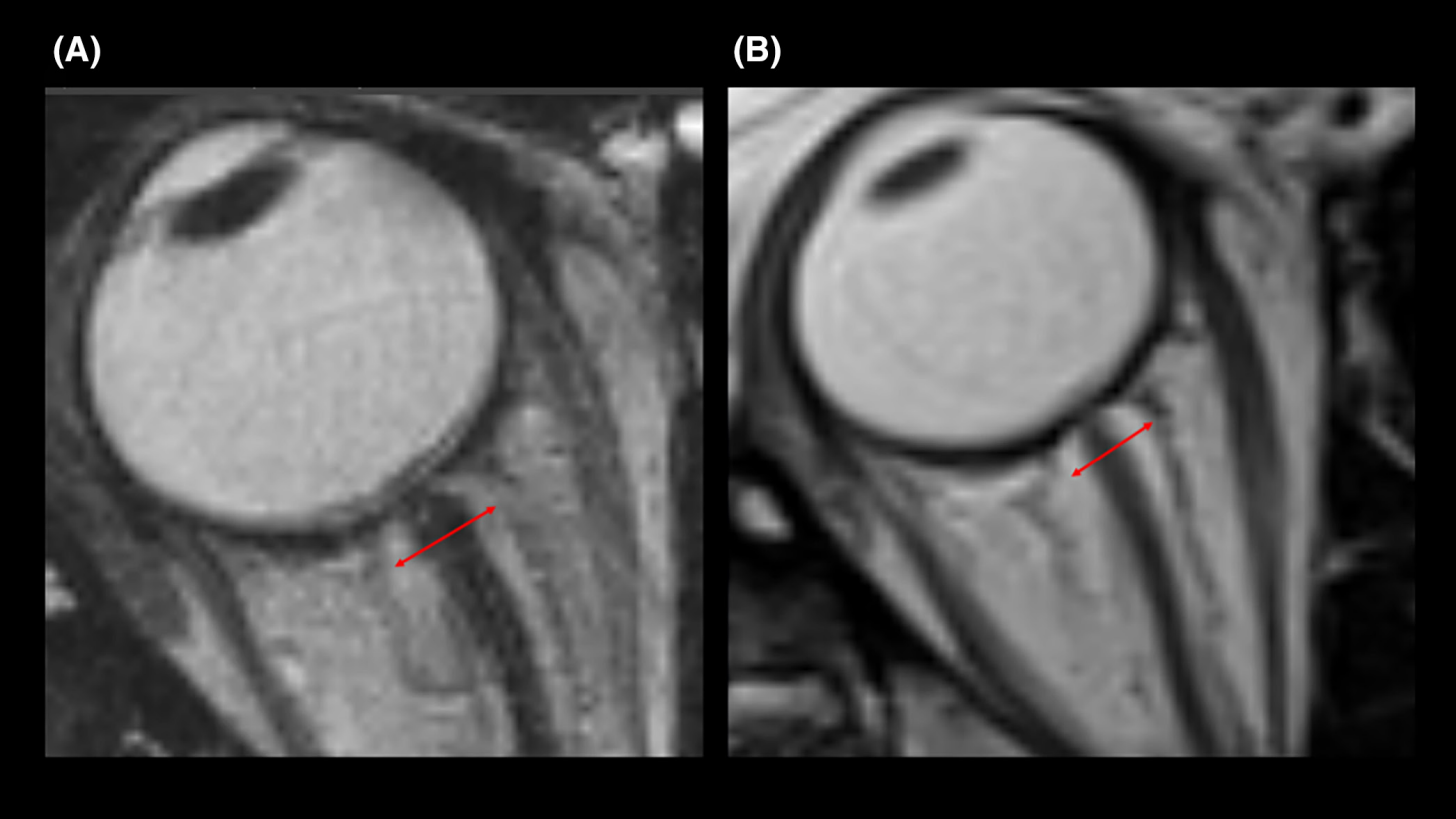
Exemplary (A) pre‐ and (B) postoperative MRI with ONSD marked red (6.4 and 5.8 mm); ONSD is measured 3 mm behind the optic disc perpendicular to the retina.
